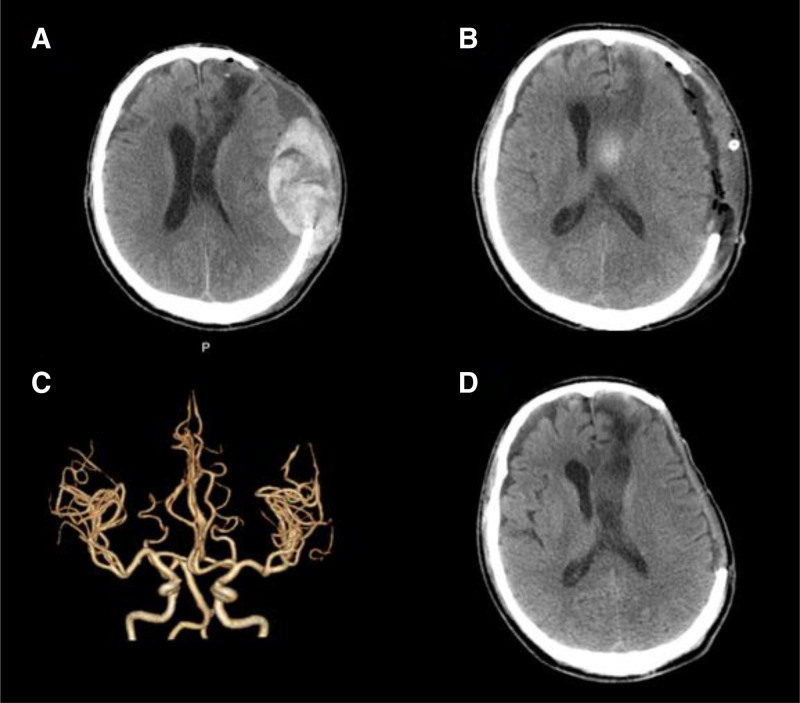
Admission and follow-up neuroimaging. (A) Cranial CT on hospital day 4: Progression of left frontotemporoparietal subdural/epidural hematomas with midline shift to the right and postoperative left cranial bone defect. The patient is currently in a coma. (B) Cranial CT on postoperative day 1: Partial resolution of left frontotemporoparietal subdural/epidural hematomas; residual hemorrhage in the left lateral ventricle, persistent midline shift, and postoperative cranial defect. (C) CTA: No significant stenosis or dilation observed in the lumens of intracranial arteries. (D) Final follow-up cranial CT: No evidence of rebleeding; minimal residual effusion in the left frontotemporal region with persistent postoperative cranial defect. Following discharge, the patient maintained clear consciousness with no reports of dizziness or headache. CT = computed tomography, CTA = computed tomography angiography.
